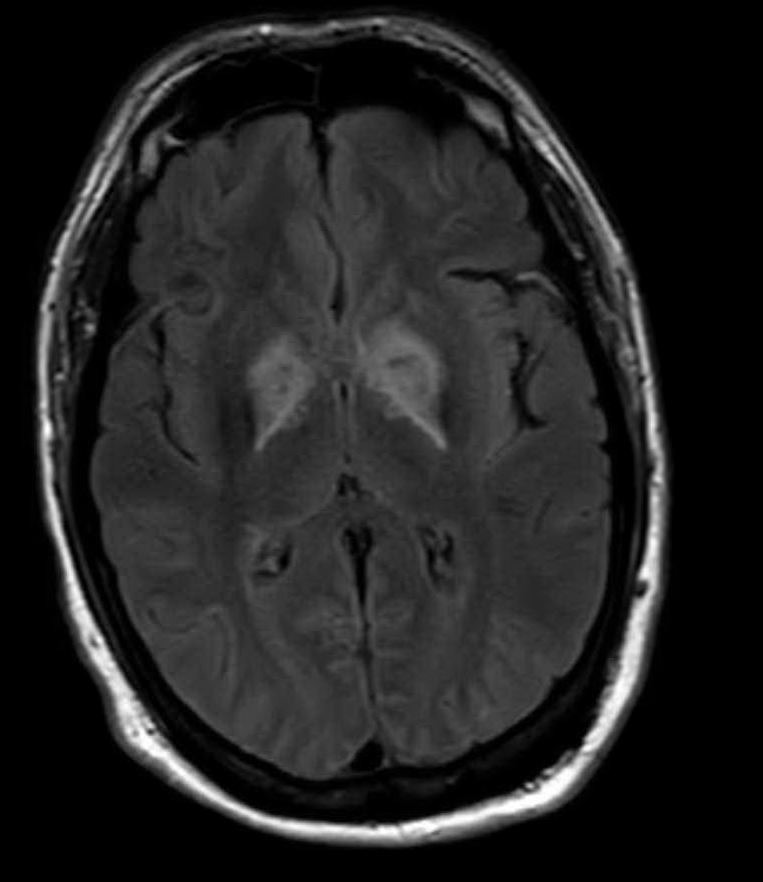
AXIAL T2 FLAIR MRI Brain Showing Hyperintense GP Relative to Surrounding Brain Parenchyma
